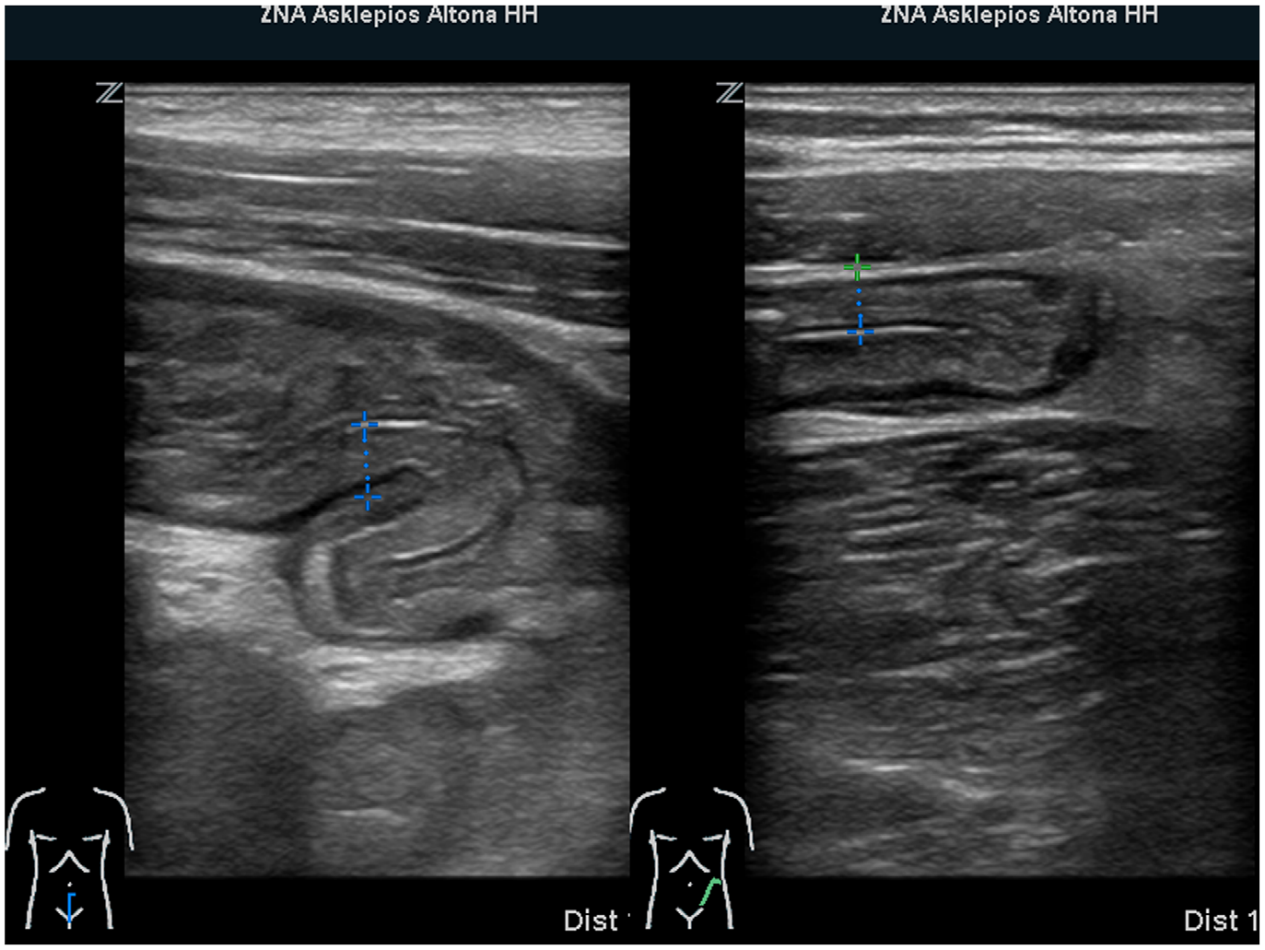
Typical ultrasound image in EHEC O104 infection. left sided colitis with marked thickening of the colonic wall.
